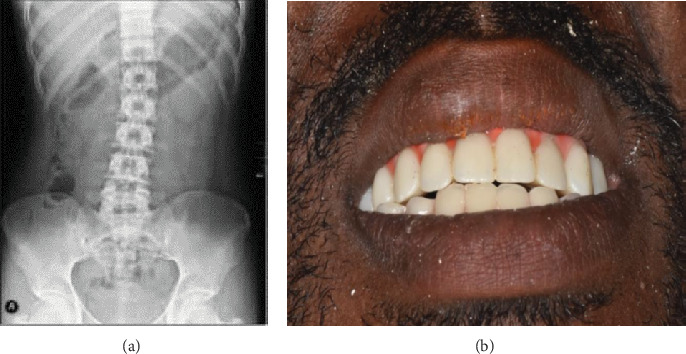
(a) An x-ray was done 2 weeks after the swallowing incident. (b) The patient after complete upper and lower jaw restorations.
